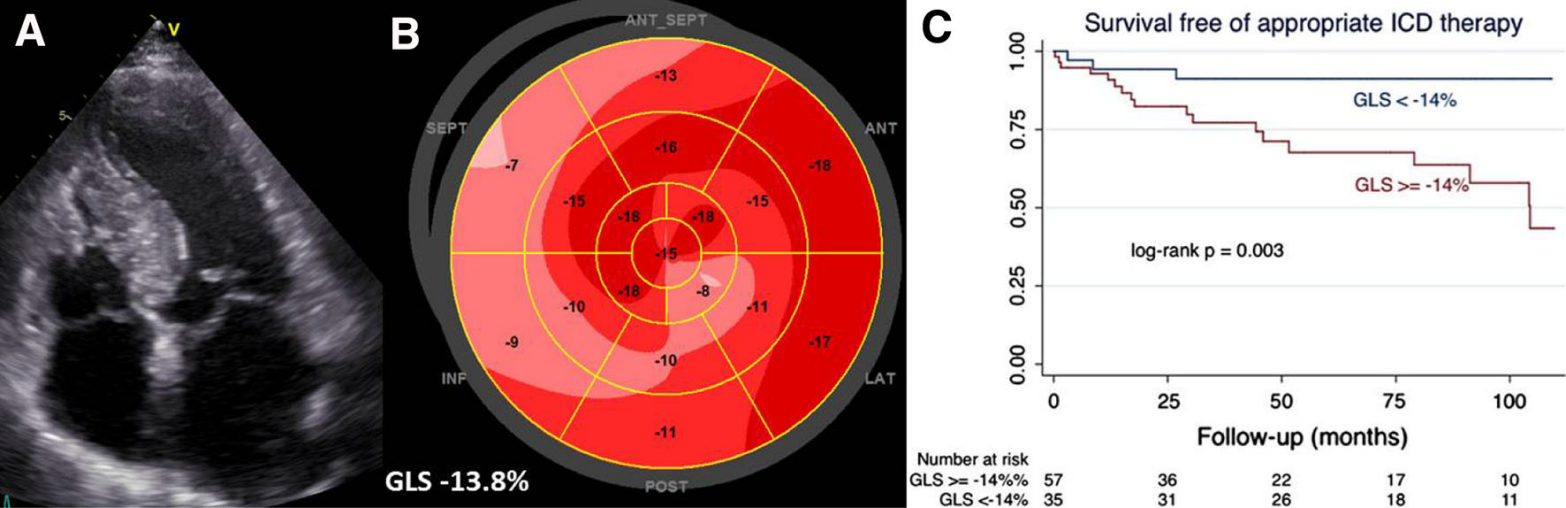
Risk stratification of patients with hypertrophic cardiomyopathy using two-dimensional speckle tracking echocardiography. Example of a patient with hypertrophic obstructive cardiomyopathy with asymmetric septal hypertrophy and systolic anterior motion of the mitral valve (A). On echocardiographic speckle tracking analysis, the magnitude of global left ventricular longitudinal strain (GLS) is −13.8% (B). The study by Debonnaire et al showed that patients with a left ventricular GLS ≥−14% had higher rates of appropriate implantable cardioverter defibrillator (ICD) therapy compared with patients with more preserved GLS (<−14%) (C). Reproduced with permission from Debonnaire et al.^61^
